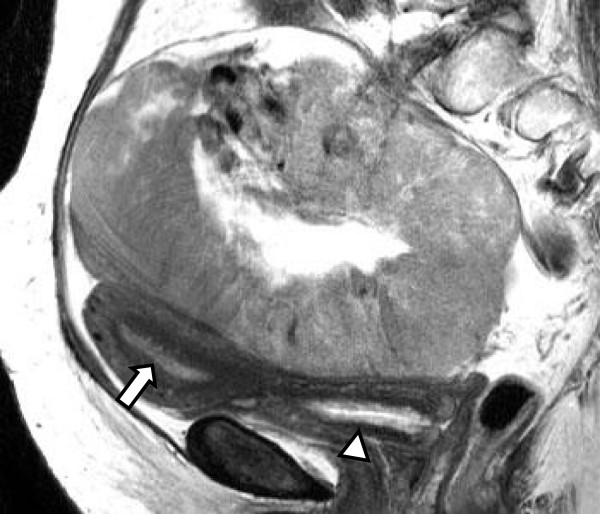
T2-enhanced MR imaging demonstrates a solid mass measuring 14 cm in the great diameter in the pelvic cavity. The uterus is found to have a thickened endometrium (arrow) and endocervical glands (arrow head) (Case 3).
